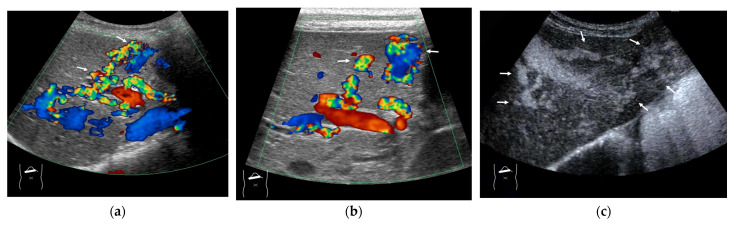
Representative case of HHT with developed A-V shunts: (a,b) Color Doppler US reveals many dilatations of HA (arrows) and vascular shunts within the liver. (c) The presence of a large A-V shunt (arrows) is confirmed using CEUS.
